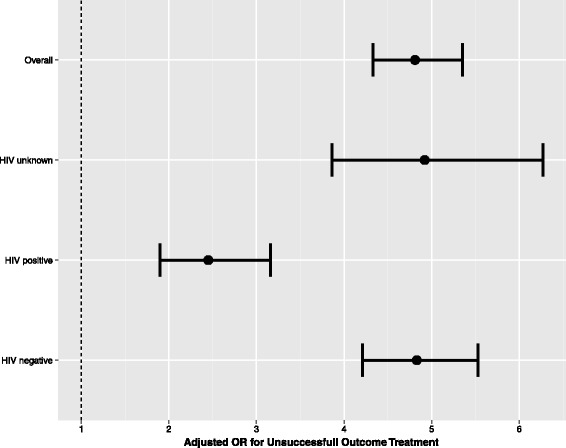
Effect modification by HIV status on the association between homelessness and the unsuccessful outcome of pulmonary TB treatment. Results from the fully adjusted model (adjusted for age, gender, country of birth, race, education level, alcohol and drug use, diabetes mellitus, mental disorder, immunosuppression other than HIV, place of diagnosis, chest x-ray and microbiologic status at diagnosis, initial drug scheme and directly observed-treatment-DOT) in the multiple imputed datasets. P value for interaction < 0.001 (Additional file 1: eTable 3)
